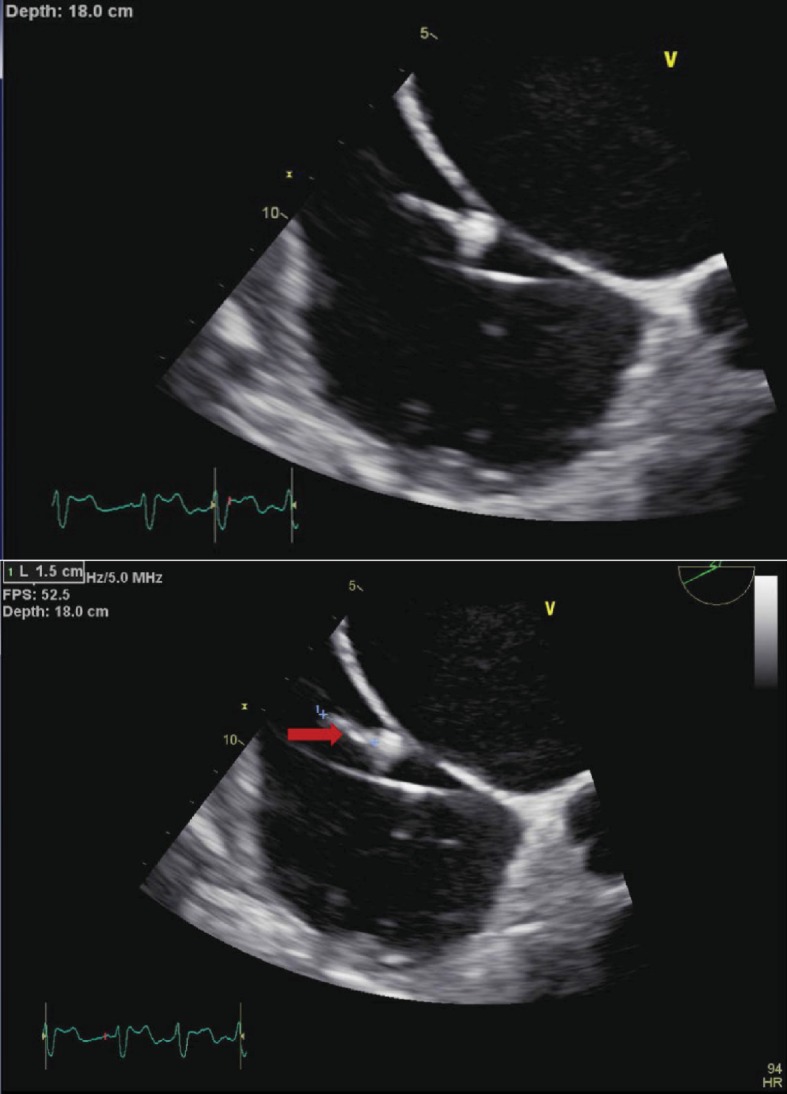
Echogenicity of one of the three leads consistent with lead vegetation measuring 0.4 × 1.3 cm. The transesophageal echocardiogram did not identify any significant valvular vegetations.
